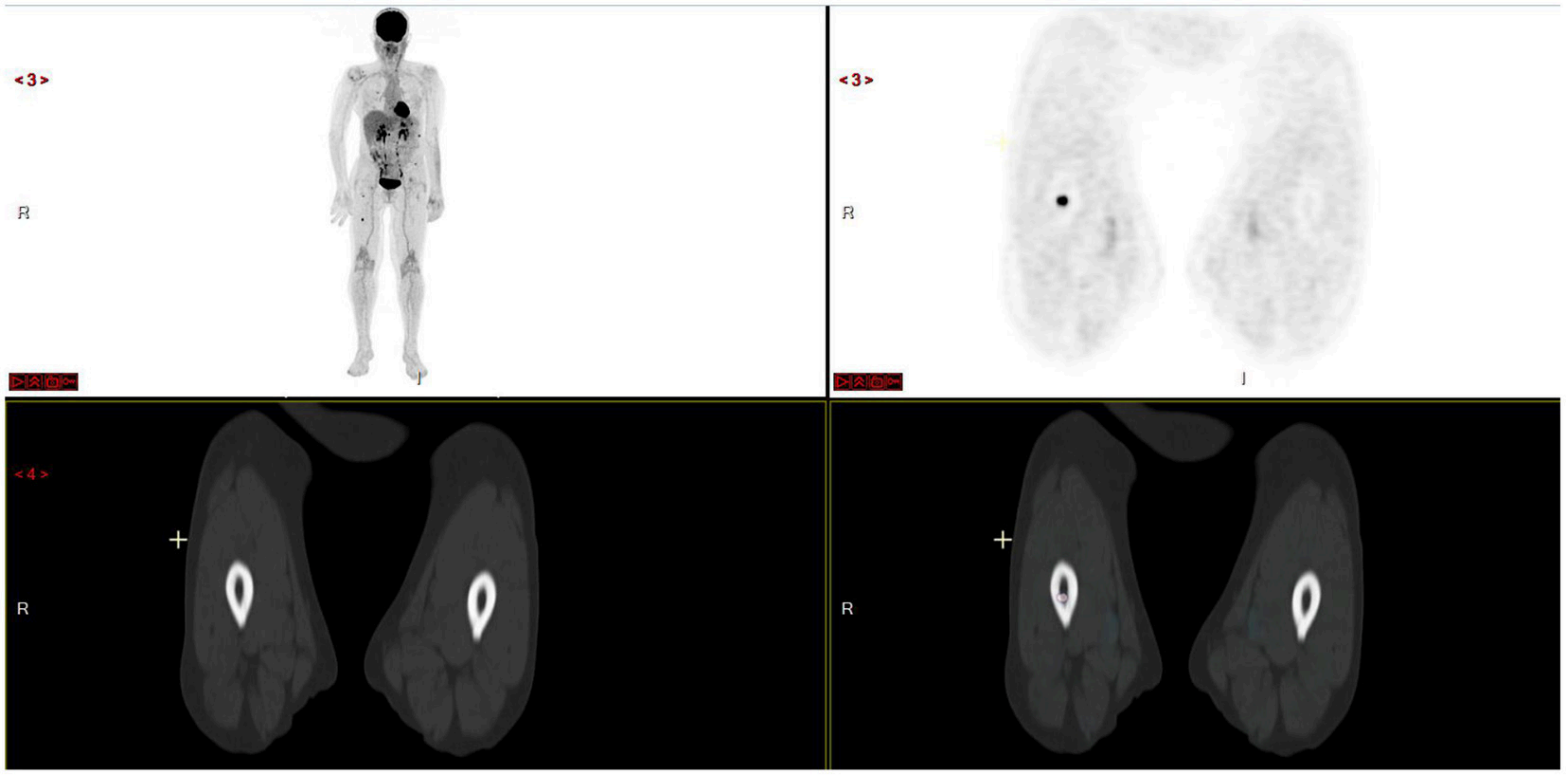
Patient with more than 10 focal lesions of the axial and appendicular skeleton. Note absence of osteolysis on opposite CT scan of right femur focal lesion.
